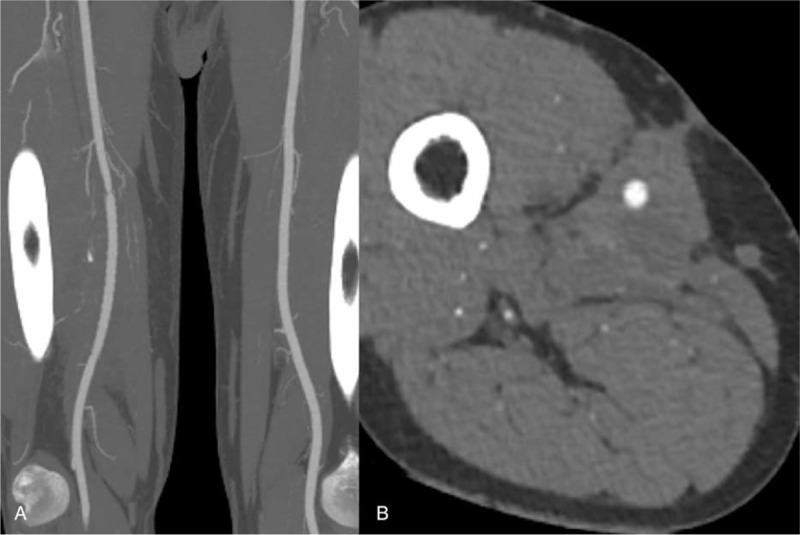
Computed tomography angiography at one-year follow-up after reintervention showes patency of prosthetic vessel and disappearance of previous hematoma.
